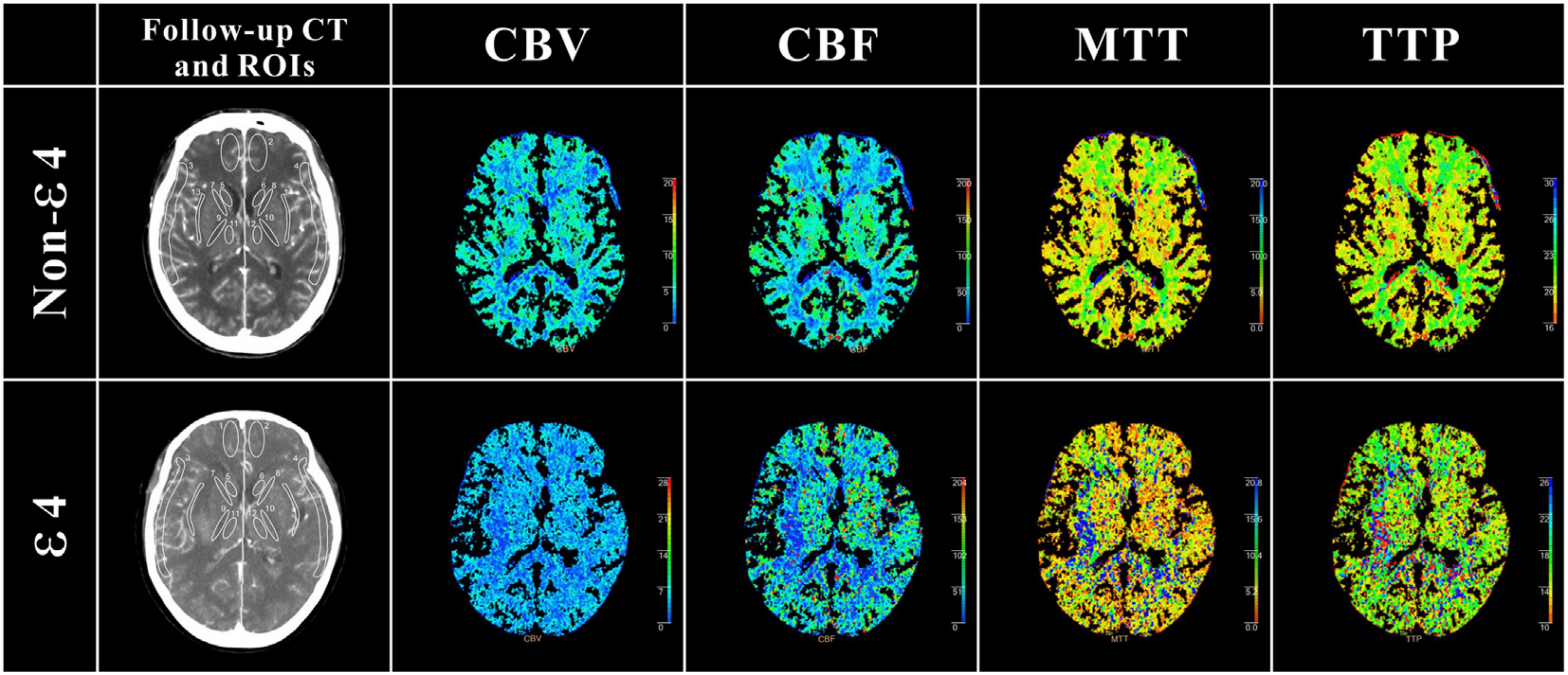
Definition of regions of interest (ROIs) in follow-up CT and cerebral perfusion images in ε4 and non-ε4 SAH patients. ROIs were drawn in 5 different regions: cortex: ROIs 1 and 2 (anterior cerebral artery), ROIs 3 and 4 (middle cerebral artery); caudate nucleus: ROIs 5 and 6; internal capsule: ROIs 7 and 8 (anterior limb), ROIs 9 and 10 (posterior limb); thalamus external: ROIs 11 and 12; capsule: ROIs 13 and 14. As shown in cerebral perfusion images, APOE ε4 allele aneurysmal subarachnoid hemorrhage patients suffered more severe perfusion disorders, especially in white matter regions.
